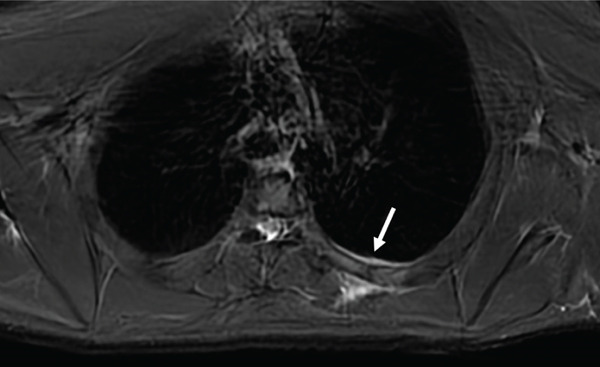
Axial T2‐weighted fat‐suppressed MR image of the thoracic wall. There is bone marrow edema at the posterior aspect of the fifth left rib, with surrounding soft tissue edema. A fracture line is seen (arrow). The finding is consistent with a BSI Fredericson Grade 4b.
